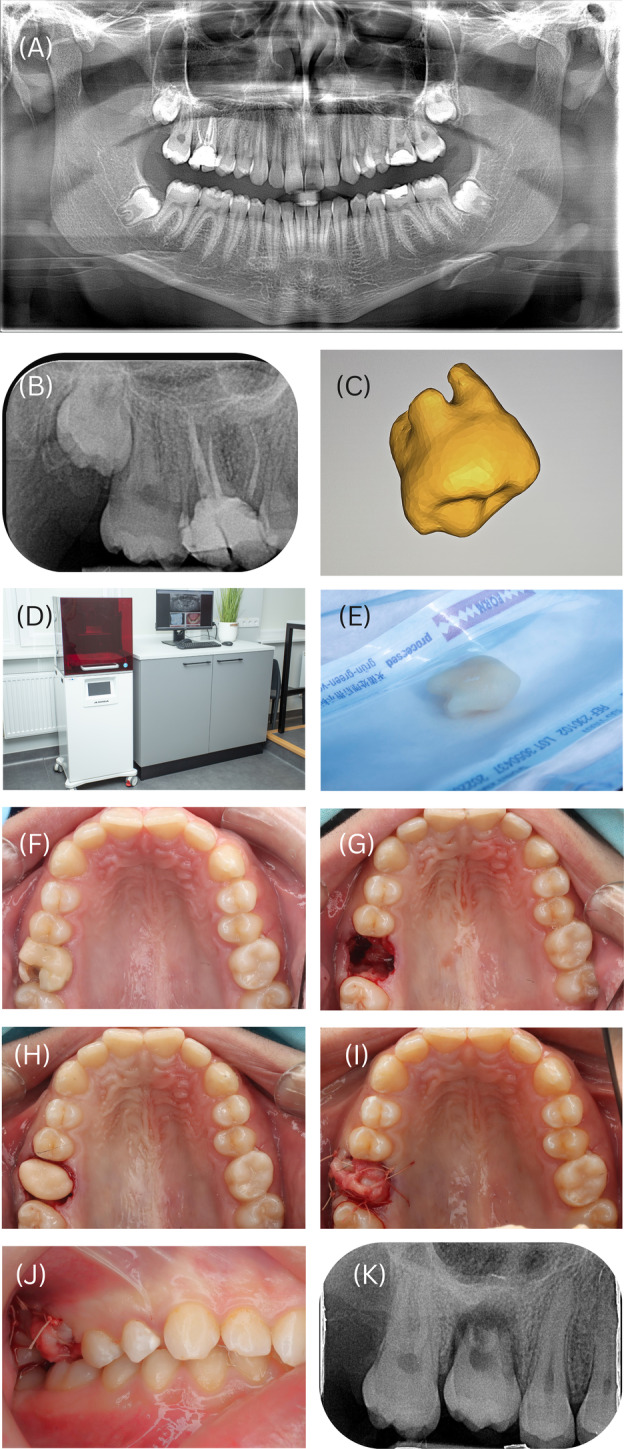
Autotransplanting an immature third molar from d18 to the d16 location. (A) Pre‐treatment orthopantomogram of a 17‐year‐old male. The upper right first molar was replaced for endodontic reasons with an unerupted upper right third molar. The donor tooth had root development at stage 3 [16] and developed 1/2 of the projected root length. (B) A periapical radiograph was obtained 1 month before surgery. (C) The 3D‐printed model was created according to the DOI:10.1111/edt.12905 protocol. (D) Asiga Max UV printer unit (Asiga, Alexandria, NSW, Australia). (E) The replica underwent sterilization at 121°C in a two‐atmosphere steam cycle, taking 60 min to complete. (F) Occlusal view before auto‐transplantation. (G) Saline‐irrigated burs were employed to prepare the recipient site after extraction. (H) Fitting of the 3D‐replica. (I) Sutures are used to stabilize the transplant. (J) Transplanted tooth test in occlusion. (K) A periapical radiograph was taken on the day of surgery.
